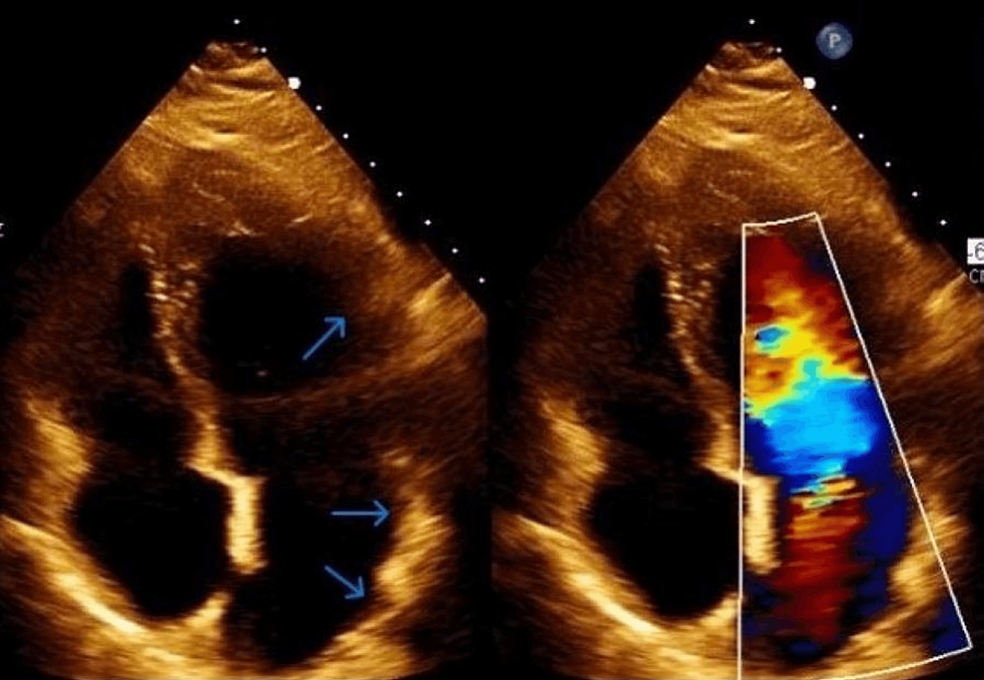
Echocardiography demonstrating multiple trabeculations (blue arrows) within the left ventricular cavity
